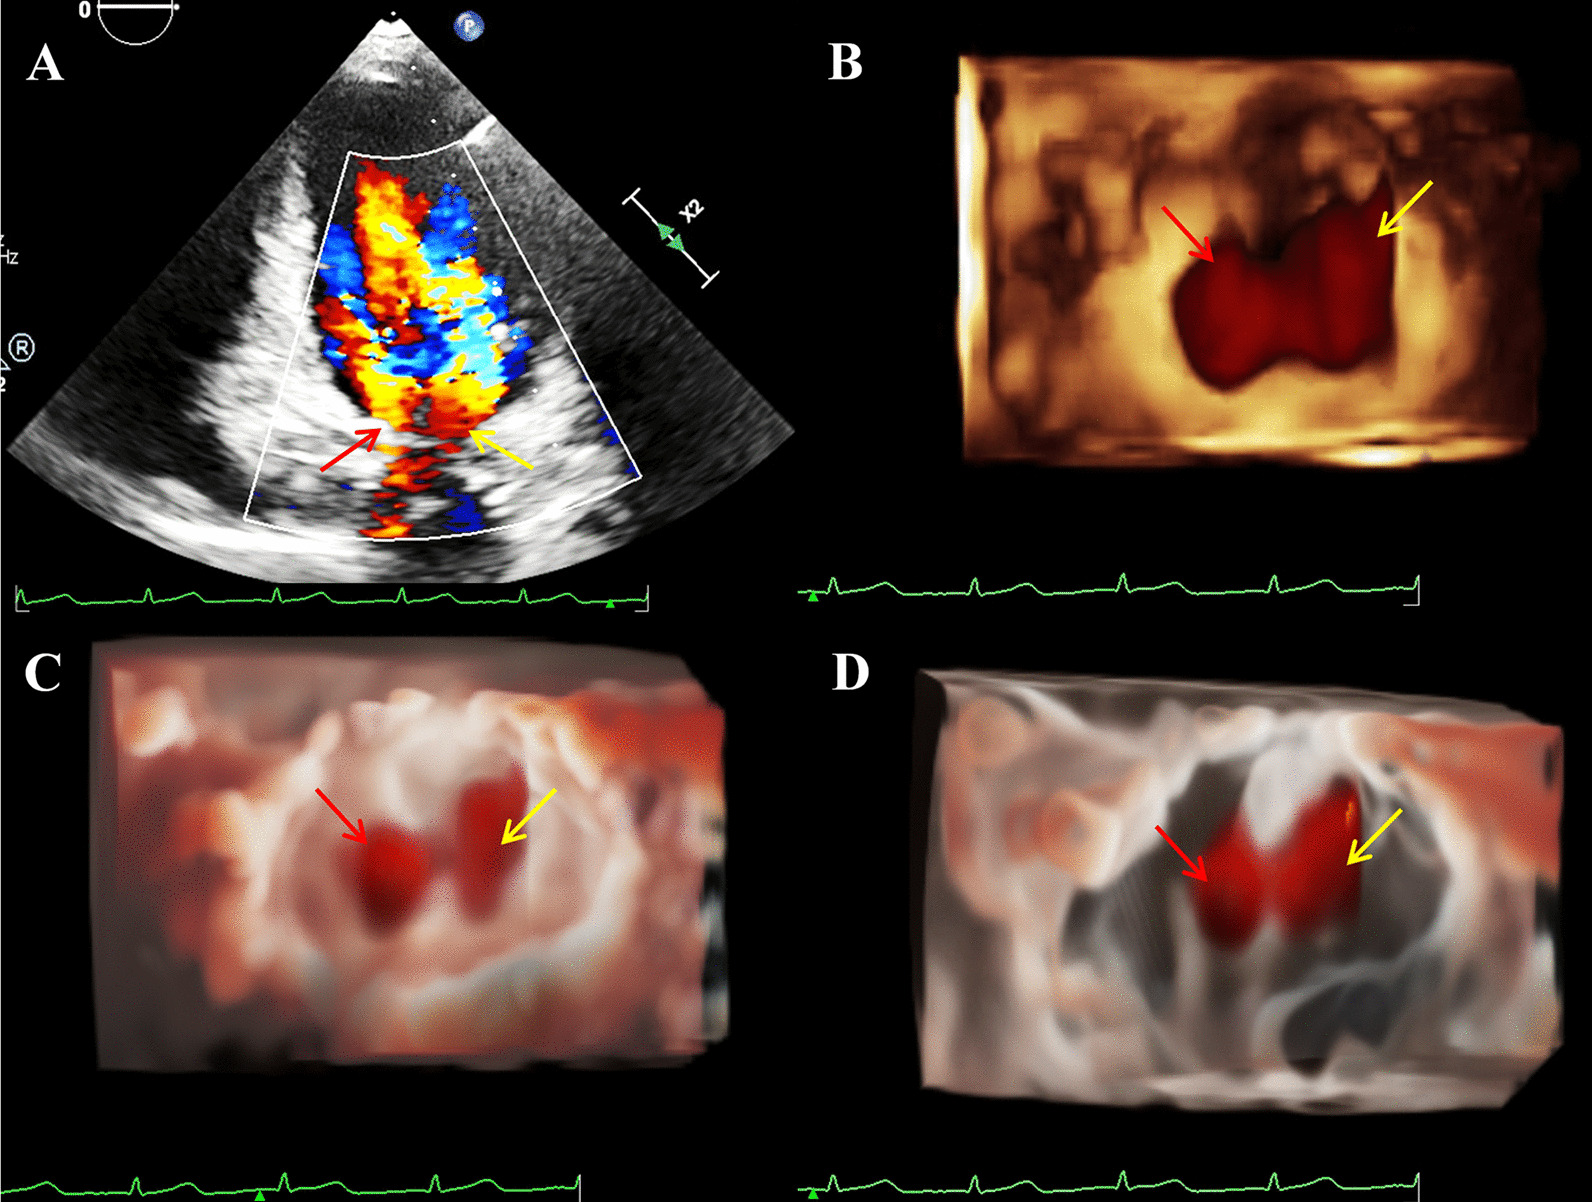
Two separate diastolic mitral inflow flows. A 2D-TTE color Doppler in the non-standard apical 4-chamber view shows that there are two blood streams flowing through the left atrioventricular channel simultaneously. B–D Traditional 3DE, TrueVue, and TrueVue Glass images showing two jets into the left ventricle. Yellow arrows show jets from orifice 1, red arrows show jets from orifice 2. *2D-TTE* two-dimensional transthoracic echocardiogram, *3DE* three-dimensional echocardiography
